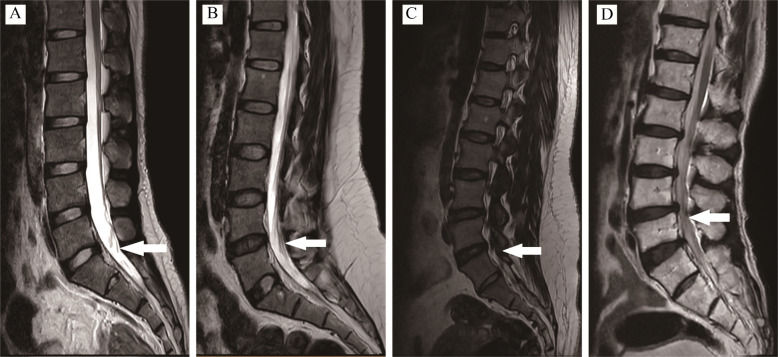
Pfirrmann grading of intervertebral discs on MRI. (A) Grade I; (B) Grade II; (C) Grade III; (D) Grade IV (White arrow)
